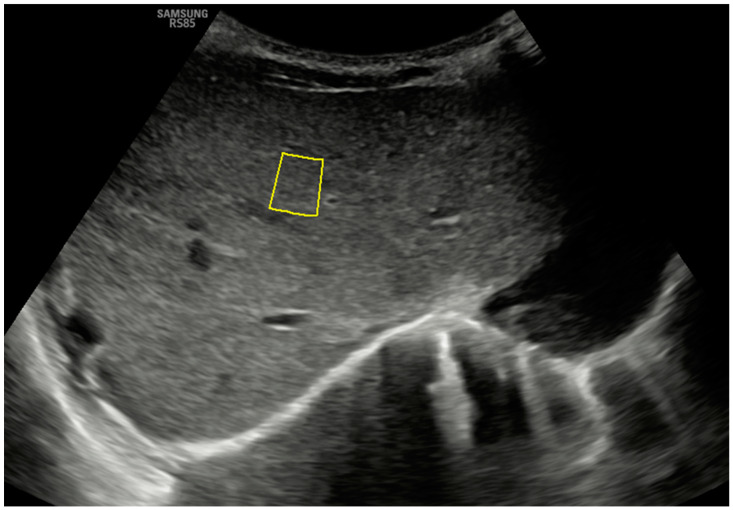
A B-mode ultrasound image in the hepatic anatomical plane focuses on segment IV and shows the correct positioning of the ROI for pSWE with a convex probe. Note the quality measurement (on this device, RMI) of 0.7.
